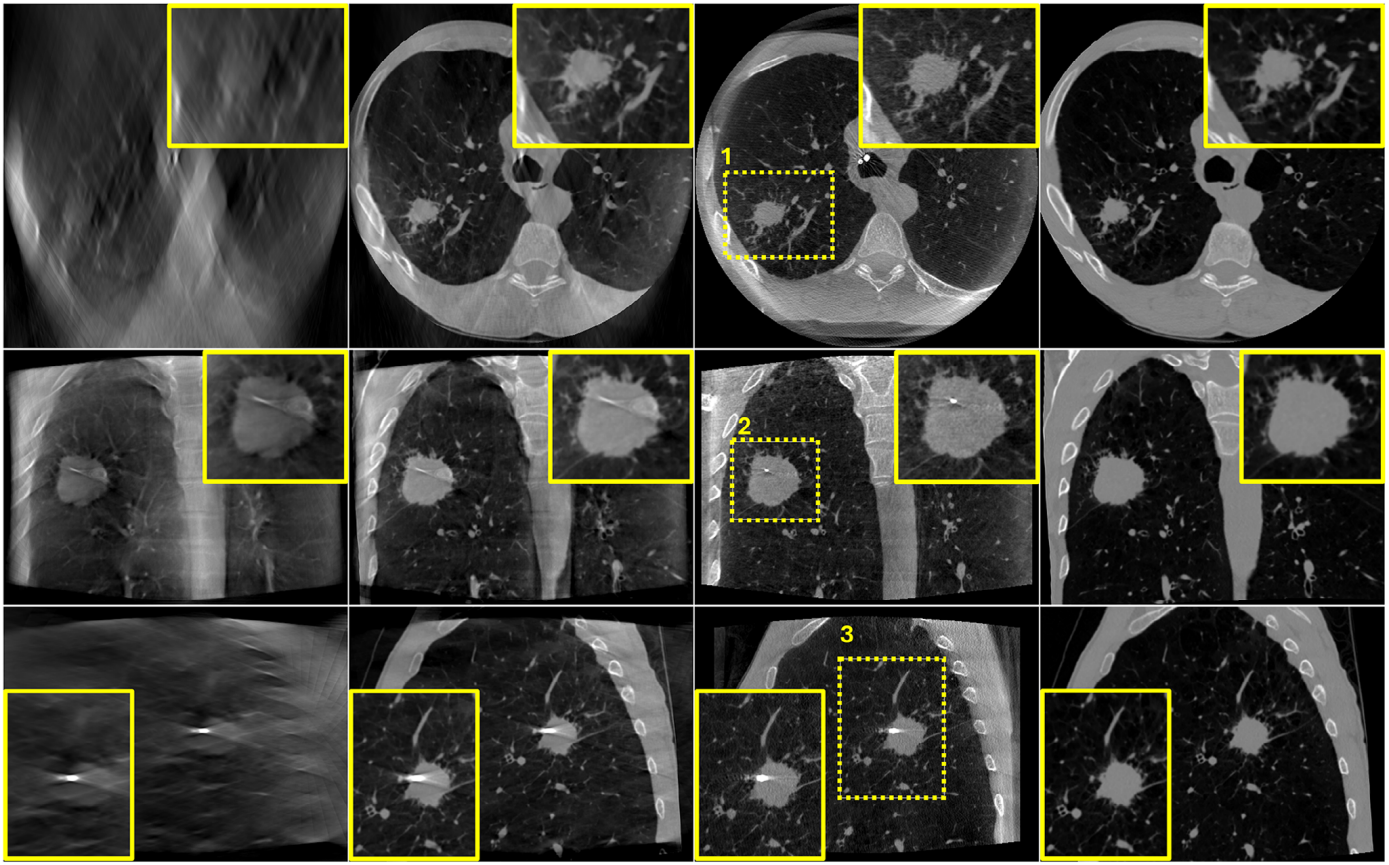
Patient bronchoscopy data reconstruction results using SE DTS data. (a) zero‐initialized ART, (b) prior‐aided reconstruction, (c) intraoperative CBCT, and (d) registered prior CT. Upper row: one axial slice. Middle row: one coronal slice. Lower row: one sagittal slice. Same slices are shown in (a), (b), (c), and (d). The display window range is [−1000 HU, 1000 HU]. ART, algebraic reconstruction technique; CBCT, cone‐beam computed tomography; CT, computed tomography; DTS, digital tomosynthesis; SE, spherical ellipse.
